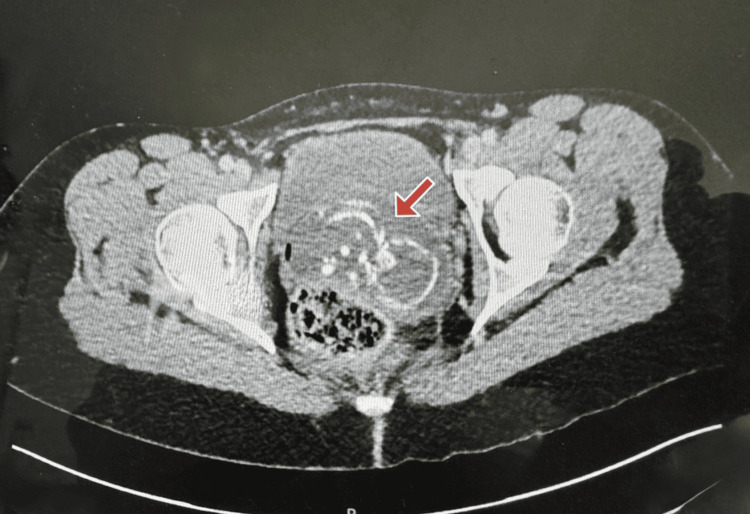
CT scan of the pelvis showing fetal bones within the uterine cavity
